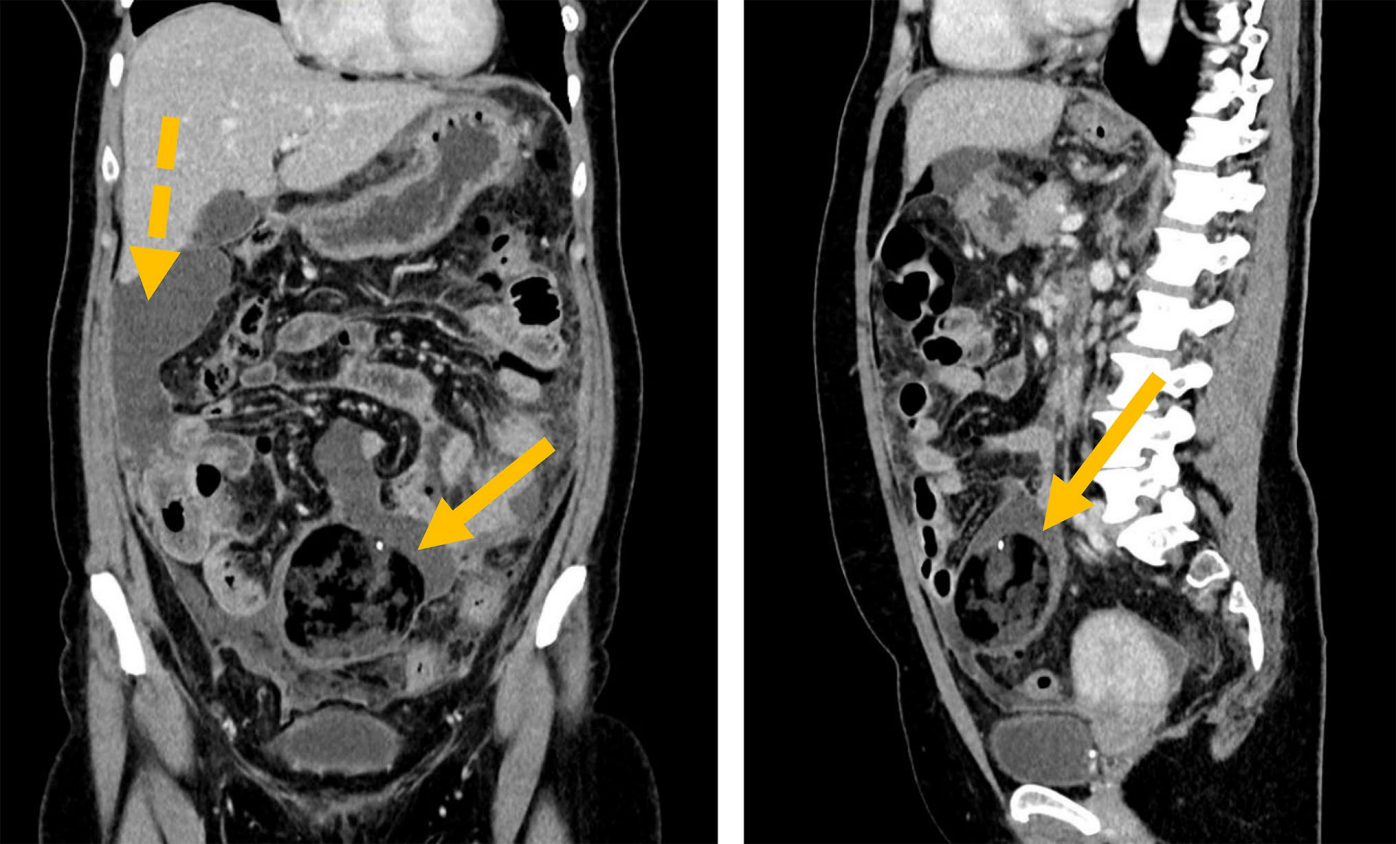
A computed tomography (CT) scan was performed at an early stage of the diagnostic process, following the ultrasound findings of perihepatic free fluids and an unclear lesion in the mid-abdomen. The solid arrow indicates the suspected teratoma, while the dashed arrow indicates the abscess-like formation
